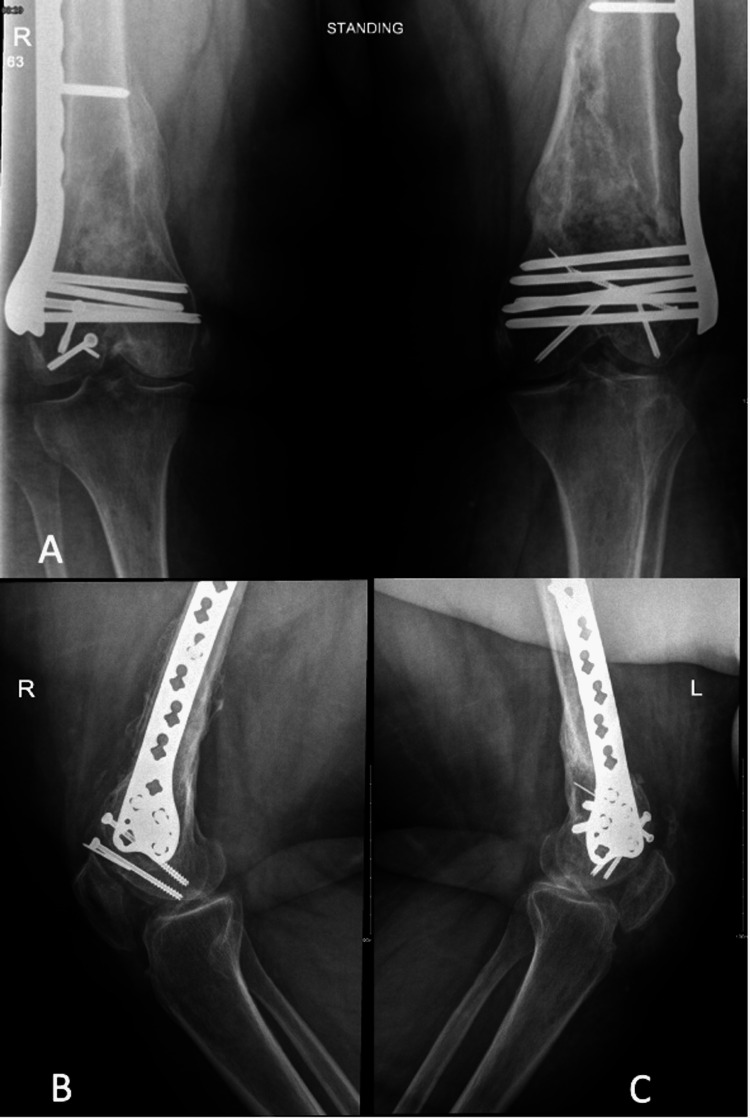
(A) Anteroposterior radiograph of the bilateral distal femur. (B) Lateral radiograph of the right distal femur; (C) Lateral radiograph of the left distal femur.
